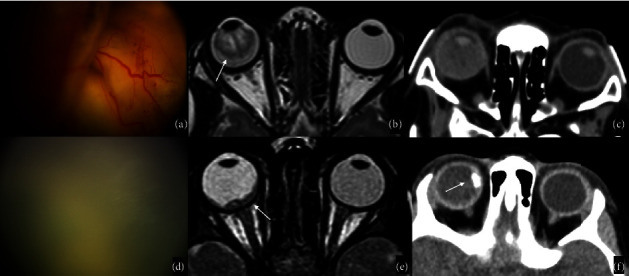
Coats Disease vs Retinoblastoma. Coats Disease (a–c). Fundus examination of a two-year-old boy presenting with exudative retinal detachment (a). An axial T2WI of the orbits confirms the presence of complete retinal detachment with vitreous hemorrhage (white arrow in (b)), while CT demonstrates the absence of ocular calcifications. Findings are consistent with Coats Disease. Retinoblastoma (d–f). Fundus examination of a three-year-old boy with diffuse vitreous seeding involving the whole vitreous cavity of the right eye. An axial high-resolution T2WI sequence shows diffuse irregular retinal thickening in the right eye (white arrow in (e)) associated with a focal calcification on CT (white arrow in (f)). Findings are consistent with the clinical suspicion of Retinoblastoma.
